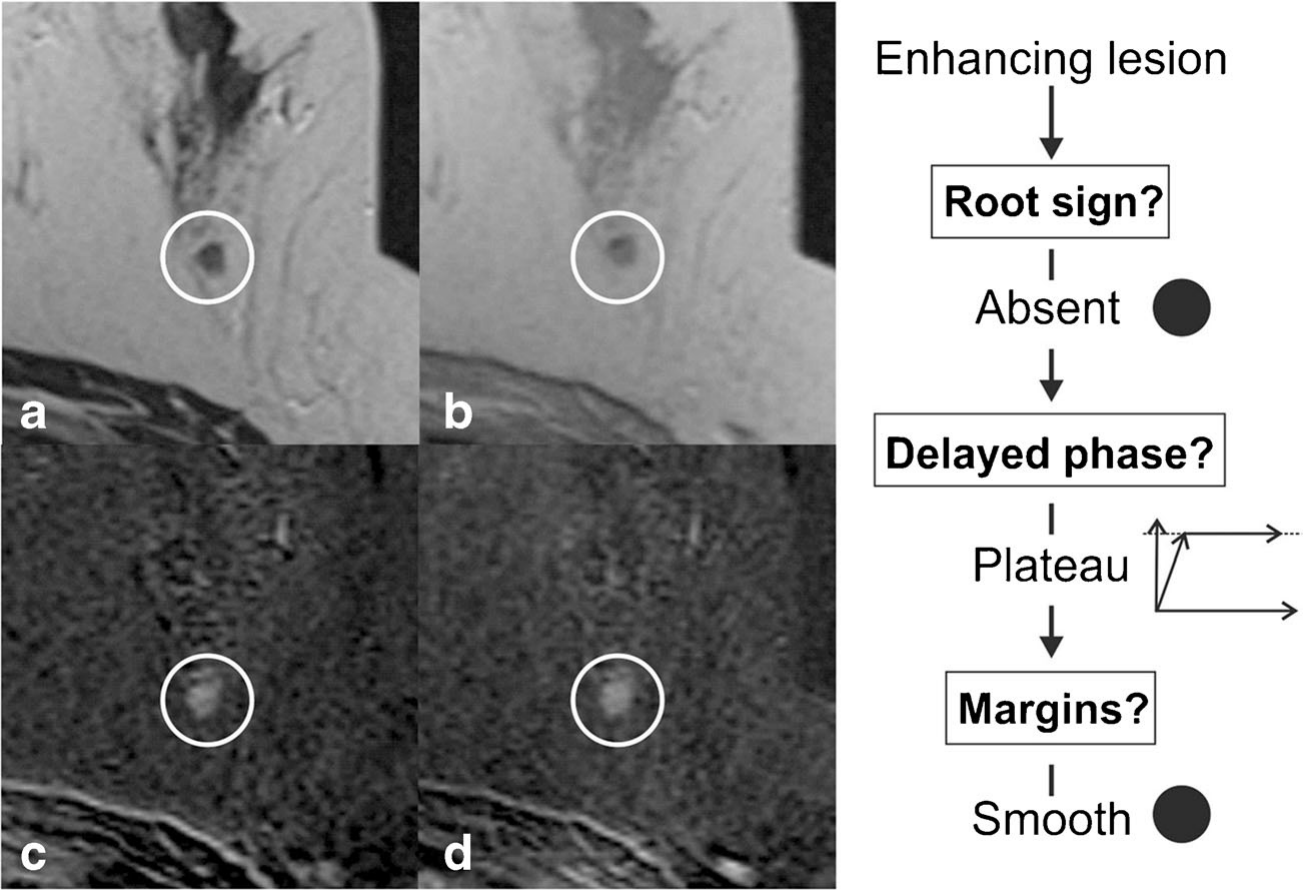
Benign columnar cell change and flat epithelial hyperplasia were diagnosed in this mass lesion showing plateau enhancement and smooth margins without the root sign, thus resulting in a *Tree* node 2. Biopsy could have been avoided with the *Tree* classification in this lesion. Representative slices of the T2-weighted (a) and the unenhanced T1-weighted sequences (b) are shown, as well as early (c) and delayed (d) subtractions of contrast-enhanced T1-weighted sequences
